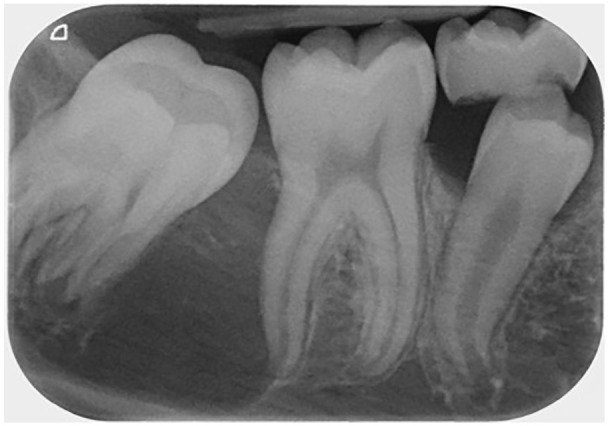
Long cone periapical radiograph of the LR6/LR7 region confirming the presence of a
